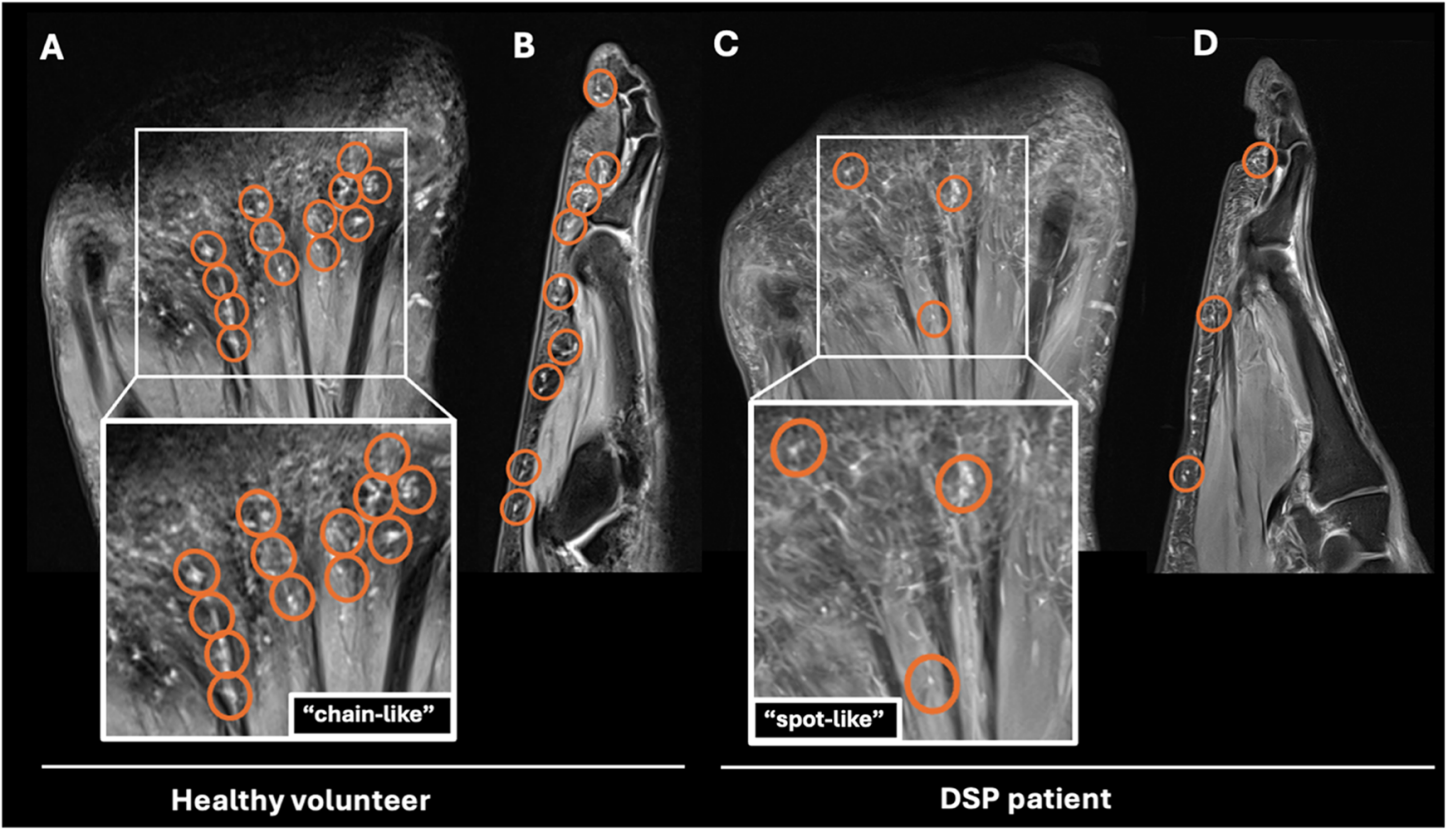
Rarefaction and disturbed arrangement of Pacinian corpuscles (PC) on forefoot MRI of a patient with diabetic sensorimotor polyneuropathy (DSP) compared to a healthy volunteer. Healthy volunteer: The coronal (A) and sagittal (at the level of the fourth digit) (B) proton-density (PD)-weighted fat-saturated (fs) images of the right forefoot of another 60-year-old healthy male volunteer depict numerous PC in the subcutaneous fat and along the deep muscle fascia (circles, A, B) showing a typical “chain-like” pattern. DSP patient: The coronal (C) and sagittal (at the level of the fourth digit) (D) PD-weighted fs images of the right forefoot of a 61-year-old male DSP patient who was diagnosed with type 2 diabetes 13 years ago demonstrate a significant rarefaction of PC numbers in the subcutaneous fat and along the deep muscle fascia and an altered arrangement with destruction of the typical “chain-like” pattern and clustering (circles, C, D). This was the predominant pattern seen in DSP patients, which we termed a “spot-like”-pattern
